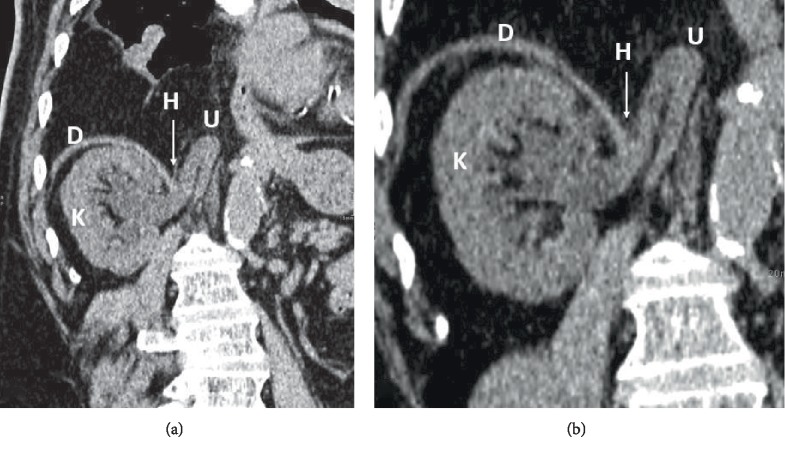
Reformatted oblique coronal images of nonenhanced CT scan of the abdomen show the right ureter herniating through a medial diaphragmatic defect causing its obstruction and secondary mild to moderate hydronephrosis. Renal cortical thickness is still preserved. D: right hemidiaphragm. K: right kidney. U: ureter. H: diaphragmatic hernia.
